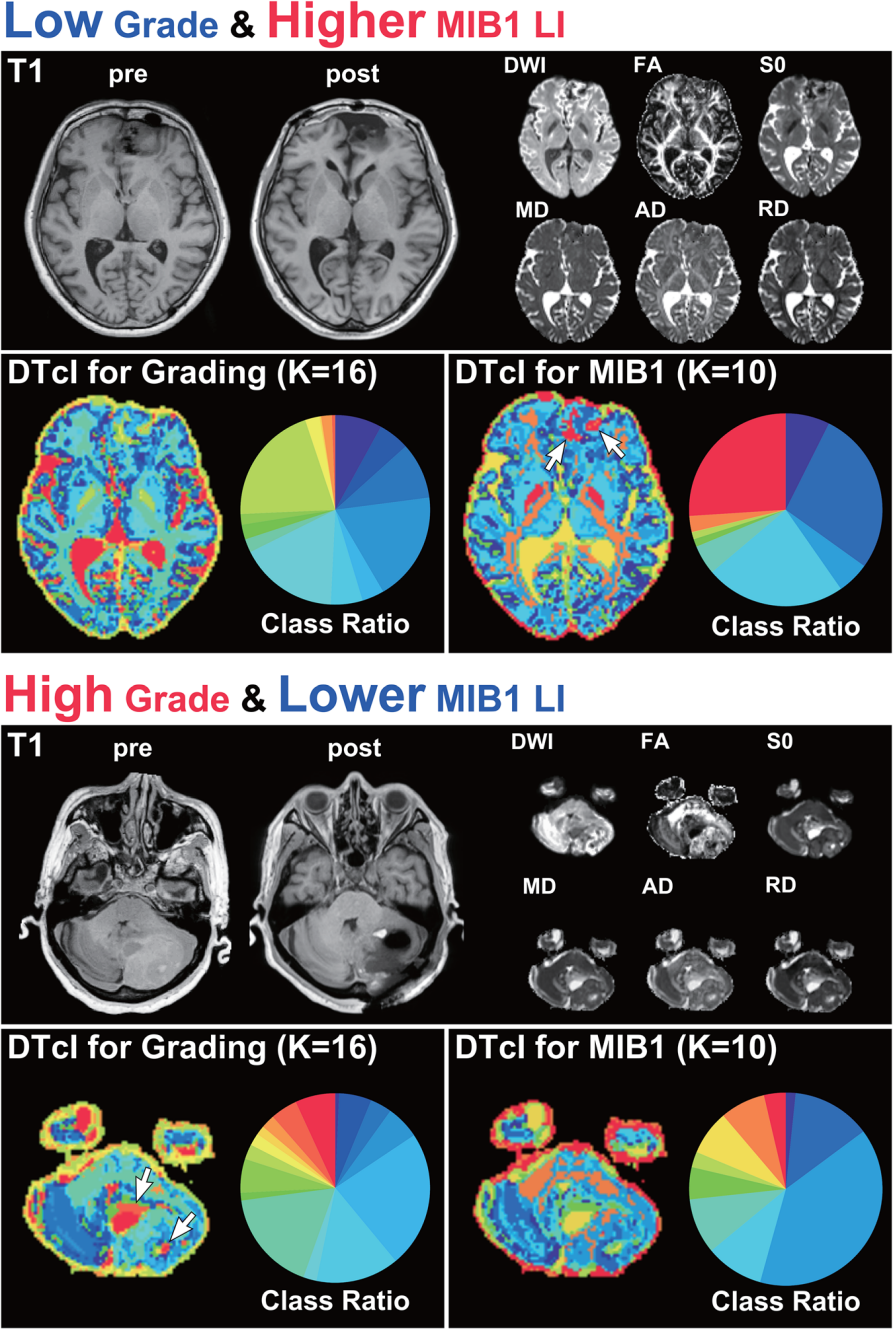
Representative cases of 16‐ and 10‐class DTcIs, which respectively showed the highest performance for classification between low‐ and high‐grade meningiomas and lower and higher MIB‐1 LIs, of a low‐grade meningioma with a higher MIB‐1 LI (upper) and a high‐grade meningioma with a lower MIB‐1 LI (lower). The upper and lower arrows show the areas of warm‐colored classes, which indicate a meningioma with higher MIB‐1 LI and a high‐grade meningioma, respectively. MIB‐1 LI, Ki‐67 monoclonal antibody labeling index; DTcI, diffusion tensor‐based clustering image; DWI, diffusion‐weighted imaging; FA, fractional anisotropy; S0, raw T2 signal without diffusion weighting; MD, mean diffusivity; AD, axial diffusivity; RD, radial diffusivity.
